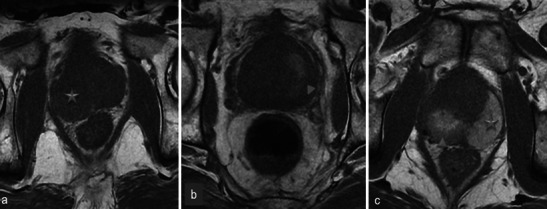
Haemorrhage within treated lobes is variable. a Day-7 post-treatment axial T1 MR image in a 64-year-old patient with right lobe photodynamic therapy shows an asymmetric prostate (*star*) with increased volume of the treated lobe but without any spontaneous high signal intensity evoking haemorrhage in the treated area. b A 72-year-old patient with left lobe treatment. Day-7 post-treatment axial T1 shows a hyperintense area (*arrowhead*) corresponding to post-treatment haemorrhagic suffusions. c Right lobe phototherapy in a 59-year-old patient. Day-7 post-treatment axial T1-weighted MR image shows a spontaneous high-signal-intensity area in the right lobe (*arrowhead*), but also an extended spontaneous high signal intensity in the left (untreated) lobe (*star*)
